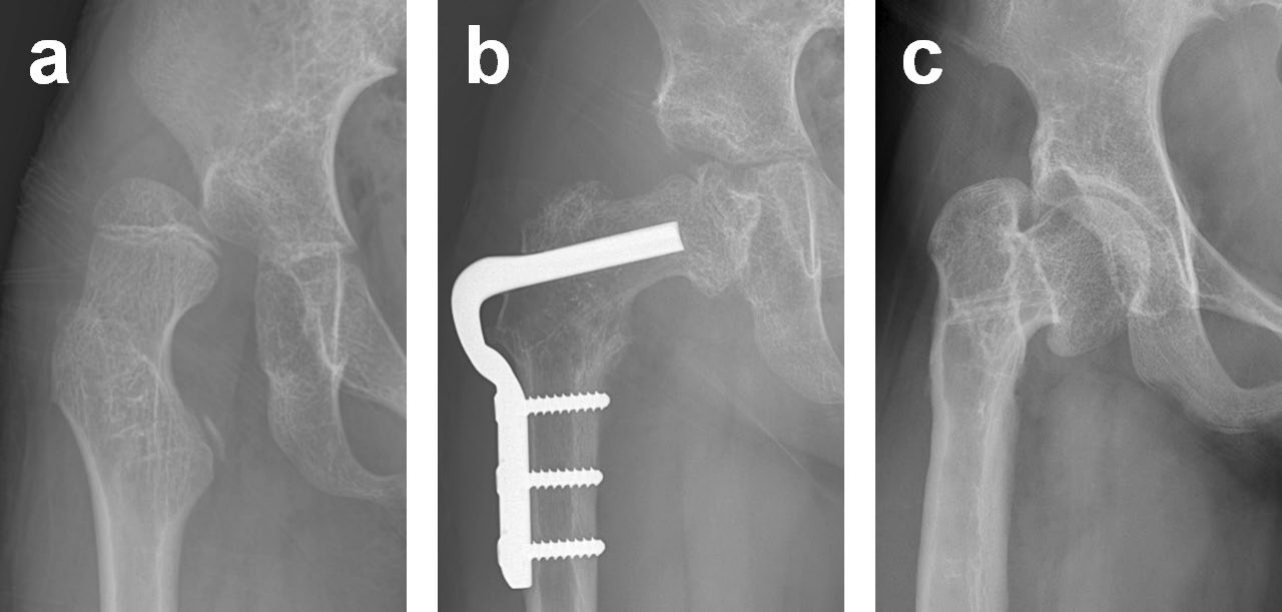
(a) Preoperative AP radiograph showing a dislocated hip in an 8-year-old girl. (b) AP radiograph showing Type 5 AVN developed after open reduction, VDRO, and pelvic osteotomy. (c) At 6 years after surgery, the femoral head was noted to be remodelled without subluxation.
